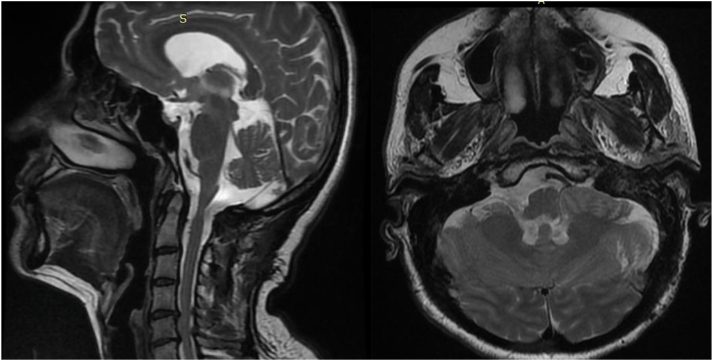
Post-operative T2 -MRI after 4 months shows no residual tumor.
